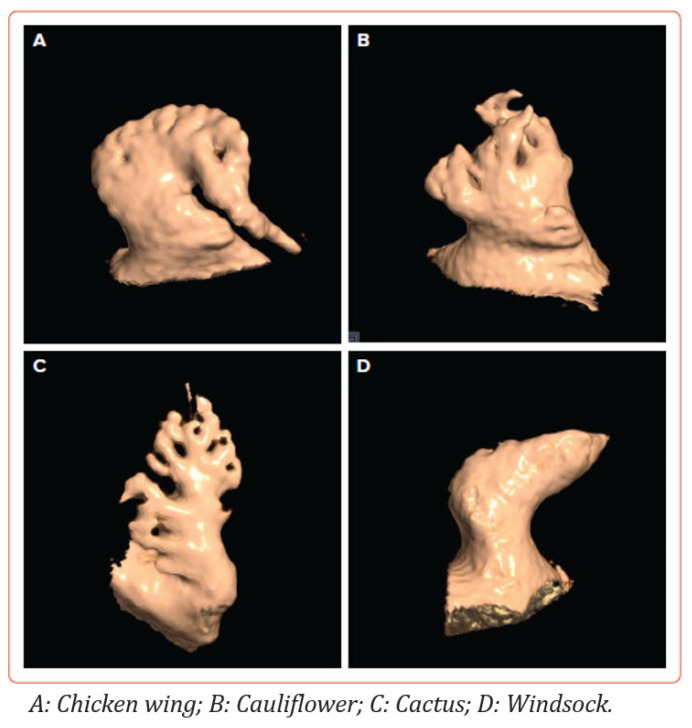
Four different left atrium appendage morphologies as shown by CT. Adapted with permission from Dudziñska-Szczerba et al. [18].
